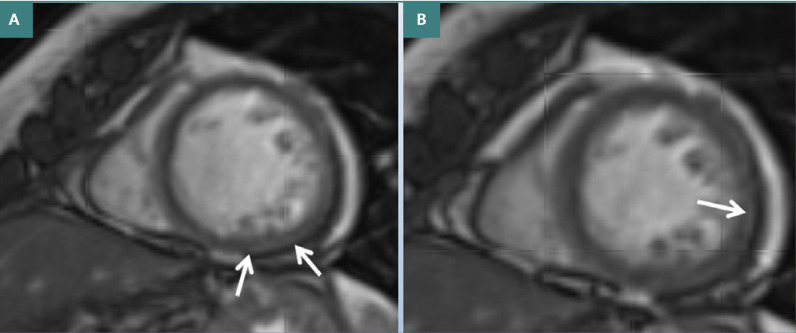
Cardiac MRI showing mild late enhancement of the posterior wall (A) and pericardial effusion of 15 mm (B), interpreted as myocarditis or myocardial fibrosis
